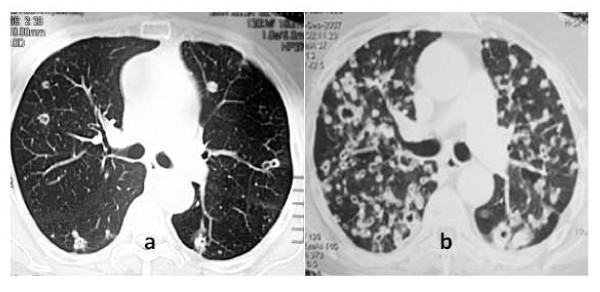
Atypical radiologic findings of excavated pulmonary metastases: initial lung CT scan (a) compared with the picture (b) after 7 months of unsuccessful chemotherapy.
